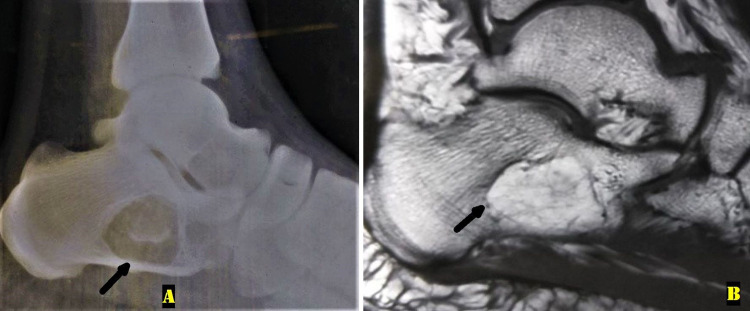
Radiologic evaluation at three years follow-up A: Plain radiograph of the right ankle lateral view showing no progression in the lytic lesion with an intact calcaneum (black arrow). B: A T1-weighted sagittal magnetic resonance image showed a non-progressive fatty lesion in the right calcaneum (black arrow), similar to the first presentation.
